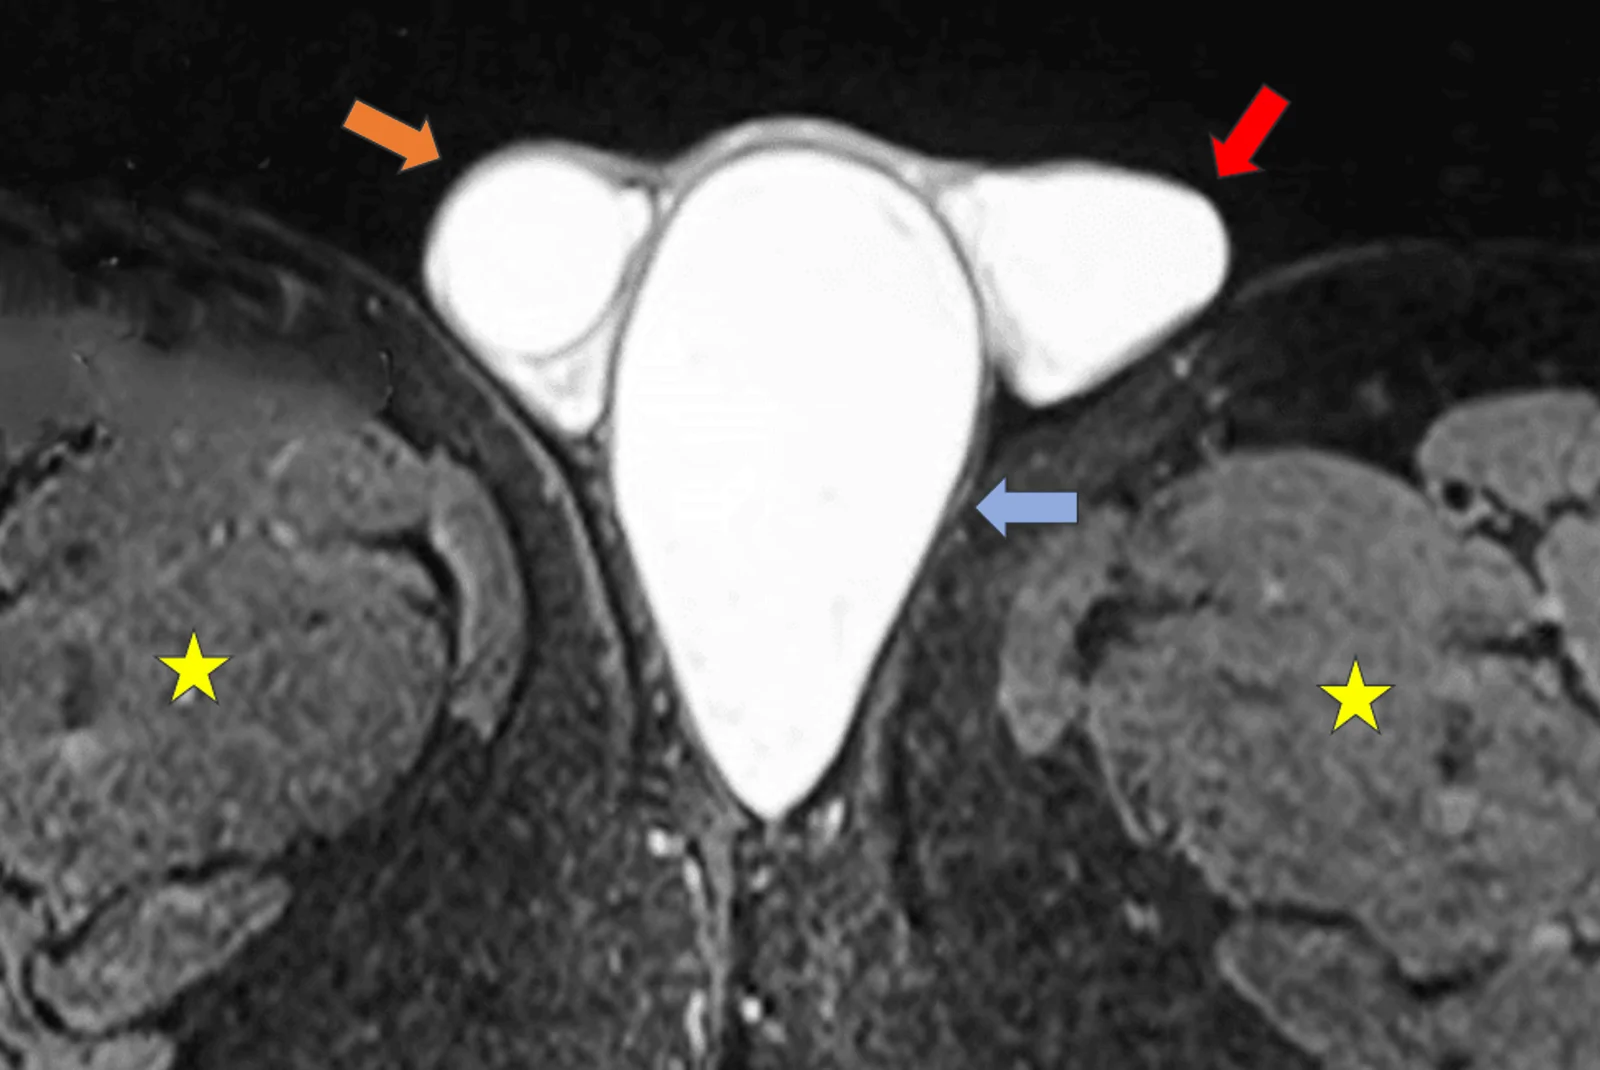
MRI image of the perineal lump in the midline, separate from the testes and surrounding muscular structures/anal sphincters Orange arrow: Right testis; Red arrow: Left testis; Blue arrow: Midline perineal swelling, appearing hyperintense on T2 -weighted imaging; Yellow star: Thigh musculature
